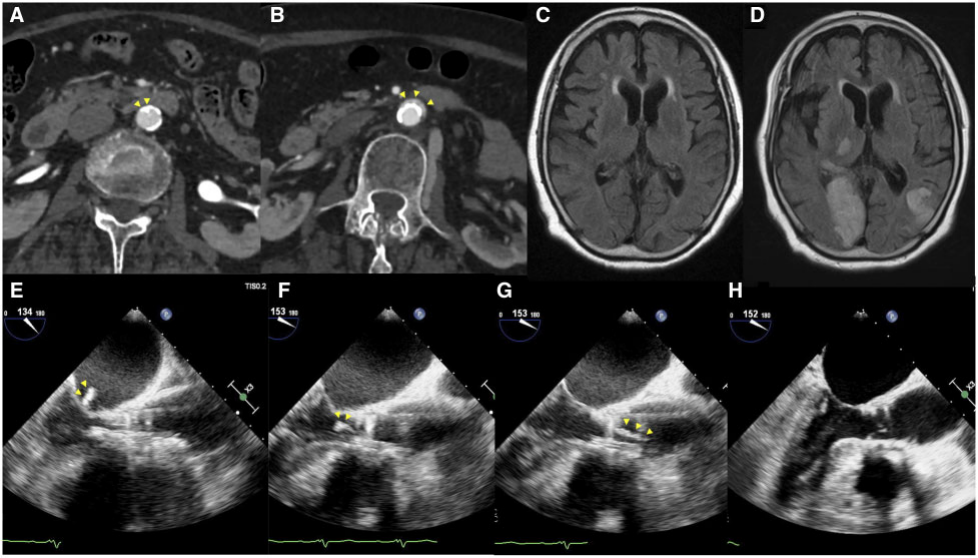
(*A*) Abdominal computed tomography prior to transcatheter aortic valve implantation. A 5 mm calcified plaque can be seen in the lumen of the abdominal aorta (arrowhead). (*B*) Abdominal computed tomography after transcatheter aortic valve implantation. Localized aortic dissection can be seen (arrowhead). (*C*) Cerebral magnetic resonance imaging prior to transcatheter aortic valve implantation. (*D*) Cerebral magnetic resonance imaging after transcatheter aortic valve implantation. Acute cerebral infarction was found on magnetic resonance imaging after transcatheter aortic valve implantation. The patient presented with hemianopsia and ataxia. (*E–H*) Transoesophageal echocardiography during transcatheter aortic valve implantation; mid-oesophageal long-axis views. A high echoic, 10 mm long mass (arrowhead) is attached to the transcatheter heart valve (*E*). Subsequently, the mass is moving in and out of the left atrium and the left ventricle (*F*). After implantation, the mass gradually enlarged to a length of 26 mm (*G*). Then, the mass spontaneously detached from the transcatheter heart valve and flowed up the ascending aorta, disappearing from the transoesophageal echocardiography field of view (*H*).
